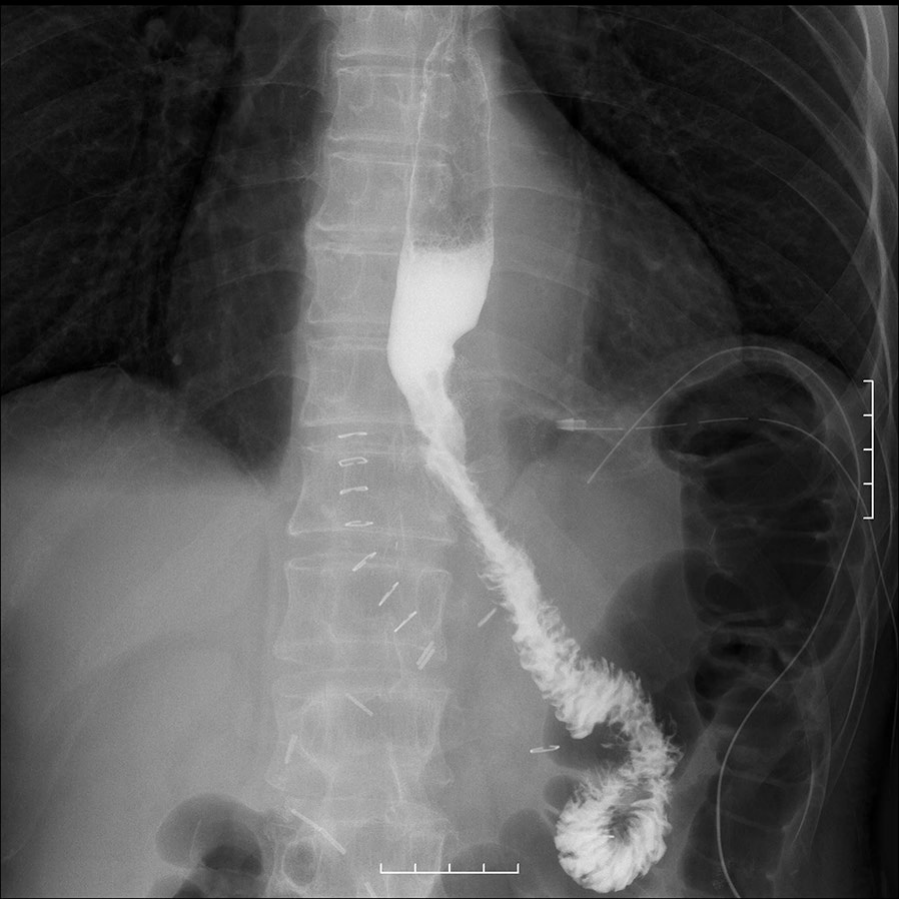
Oral contrast radiography 4 days after re-anastomosis. The outflow of contrast medium was good, and no stenosis or reflux occurred
